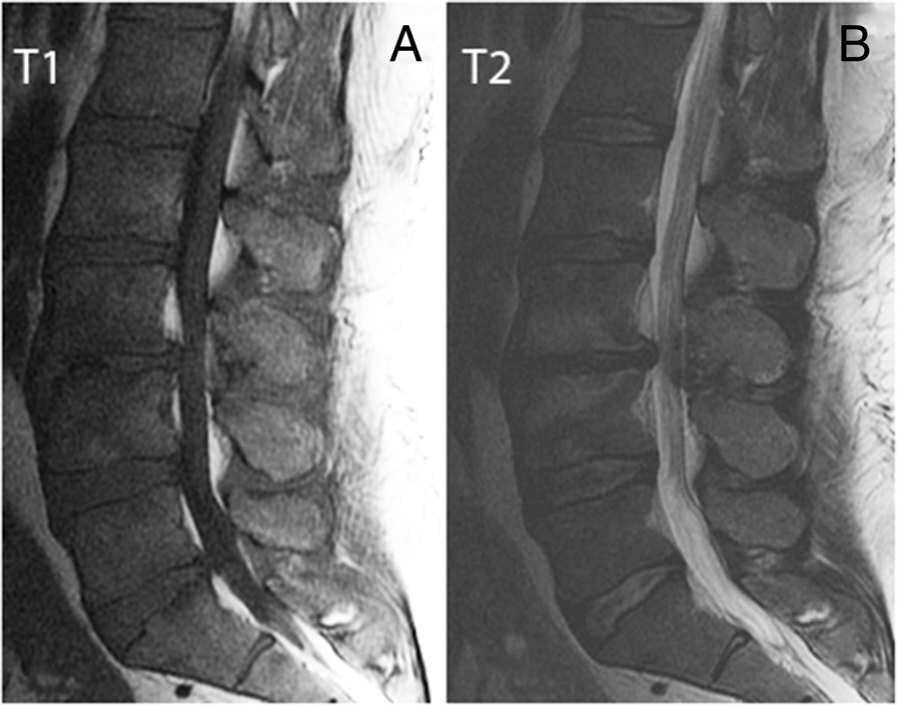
Comparison of the sacral area on T1 and T2 sagittal images. (A) The T1 on the left shows the thecal sac is partially obscured by EL. (B) The T2 sagittal MR image on the right shows a clear outline of the thecal sac ending at mid S2.
